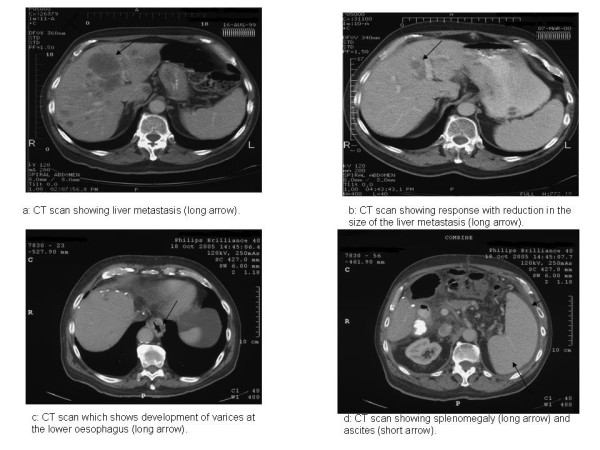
CT scans. (a) Liver metastasis (long arrow). (b) Response with reduction in the size of the liver metastasis (long arrow). (c) Development of varices at the lower oesophagus (long arrow). (d) Splenomegaly (long arrow) and ascites (short arrow).
